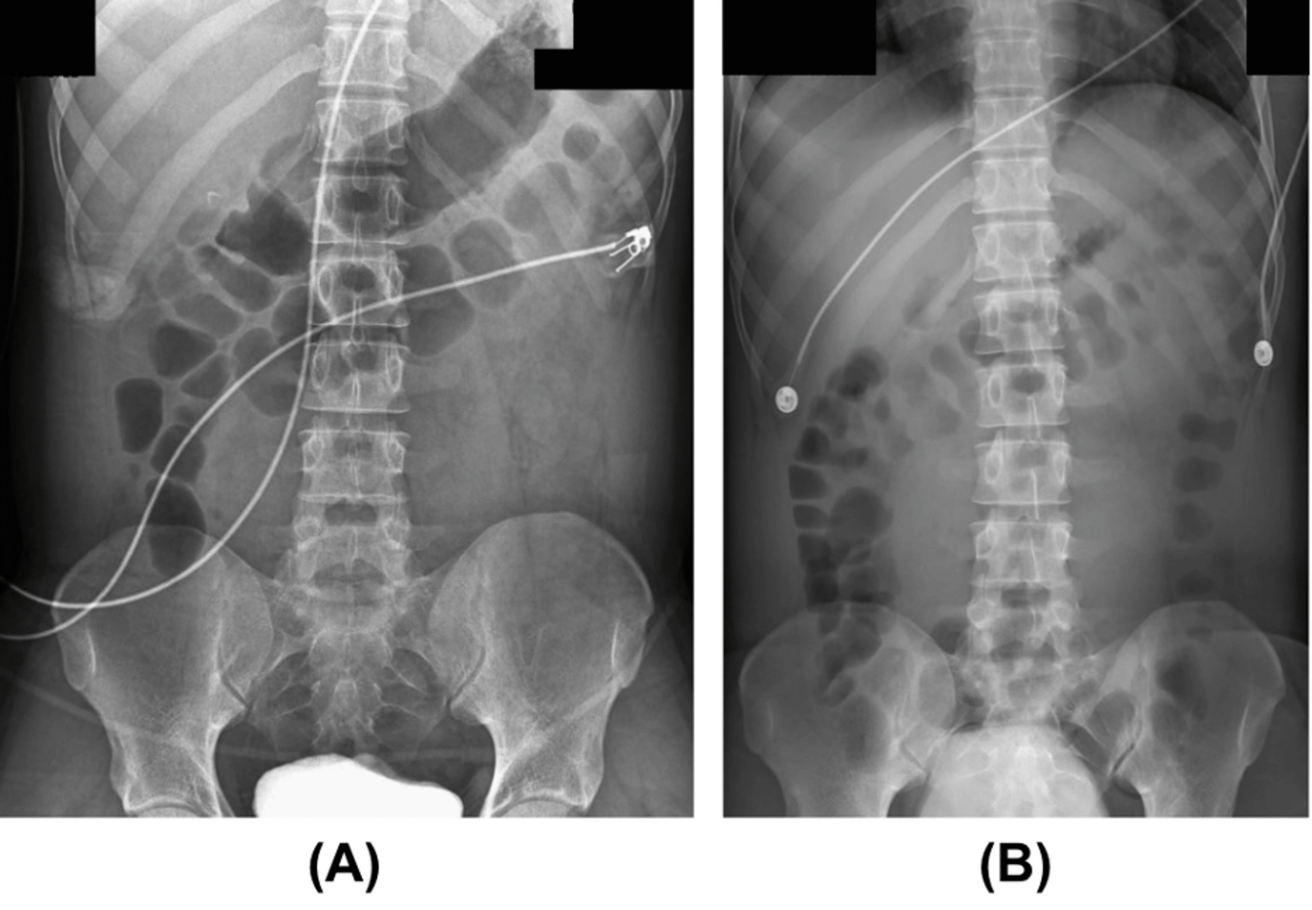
Abdominal X-rays demonstrating evolution of suspected small bowel obstruction (SBO). (A) An abdominal X-ray on the day of admission was significant for a possible SBO due to the presence of dilated small bowel. (B) A repeat abdominal X-ray on hospital day two without significant small bowel dilation signified that the patient did not have an SBO during this admission.
